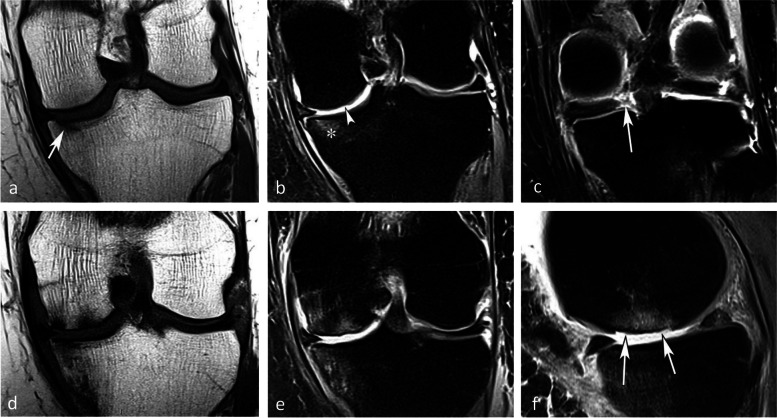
Pejorative evolution of SIF related to cartilage degradation. a, b Initial T1-weighted MR image shows a typical subchondral insufficiency fracture in the medial tibial plateau on T1-weighted image (arrow in a) with a BME-like high-signal intensity on fat-suppressed T2-weighted image (asterisk in b). c The lesion is associated with a radial tear of the posterior horn of the medial meniscus (arrow). d–f Six months later, T1-weighted (d) and fat-suppressed T2-weighted (e) images show various signal intensities in bone on both sides of a severe chondral loss (arrows in f). The initial examination already showed a clear thinning of the cartilage of the medial condyle (arrowhead in b), which could have indicated a pejorative evolution
